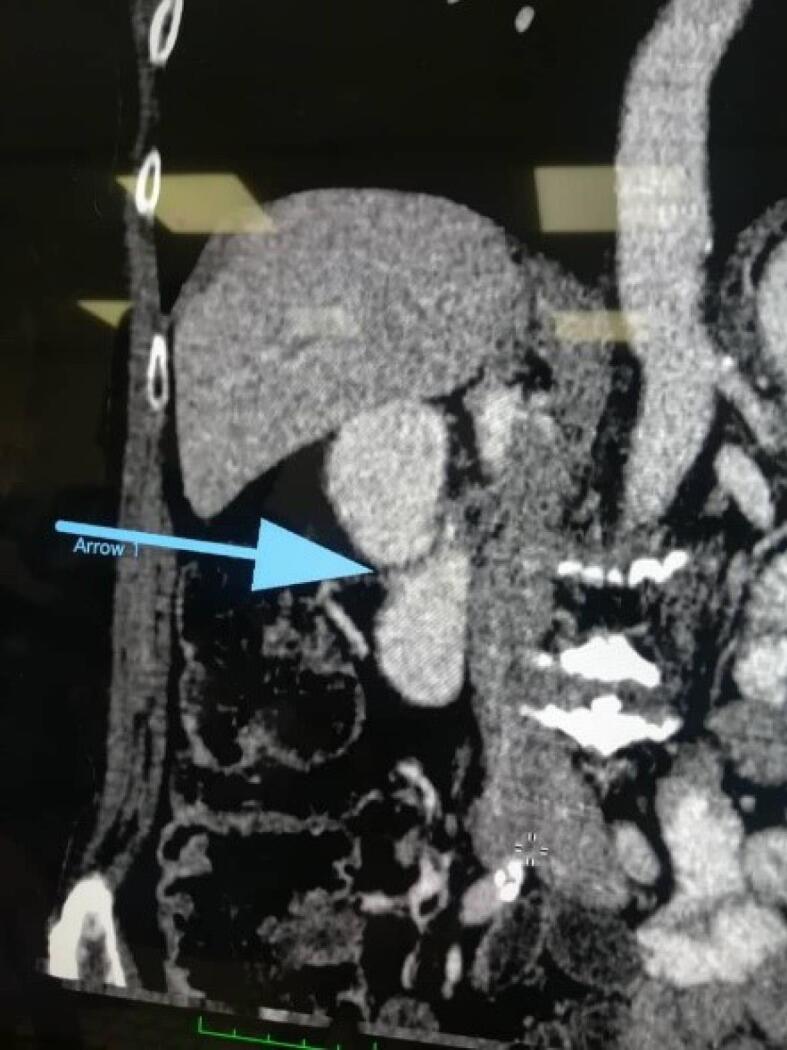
Finally, to start the treatment process, the patient was referred for surgical exploration. Considering the patient’s age, preoperative echocardiography was undertaken, and the ejection fraction was 55% without any abnormality. After initial assessment and hemodynamic stabilization, the patient underwent laparotomy and gastrojejunostomy. On the third postoperative day, the patient was allowed oral intake, starting with clear liquids, which were well tolerated. After the operation, the patient had no problem with defecation and was also able to take a full mixed diet by the seventh postoperative day. Finally, the patient was discharged in good general condition with no complaints of symptoms.
